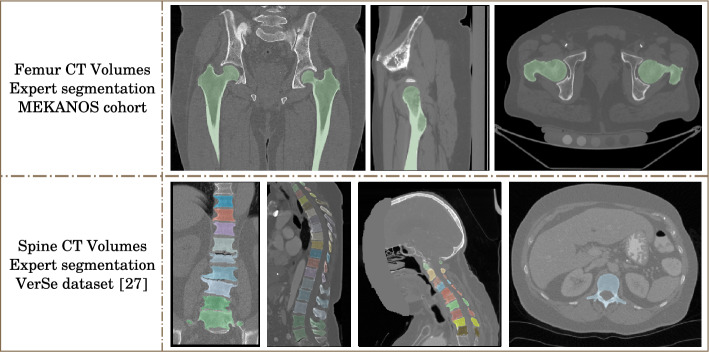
Example of CT data used for vertebrae and femurs segmentation.
